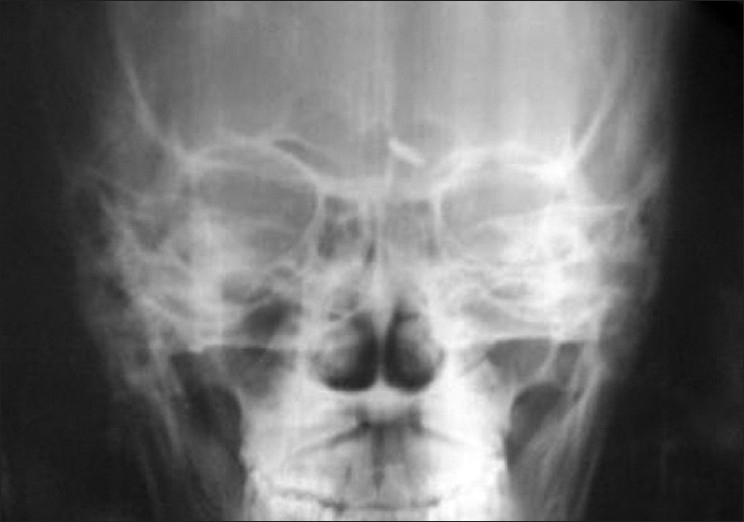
X-ray of the skull showing persisting splinter in the frontal area
